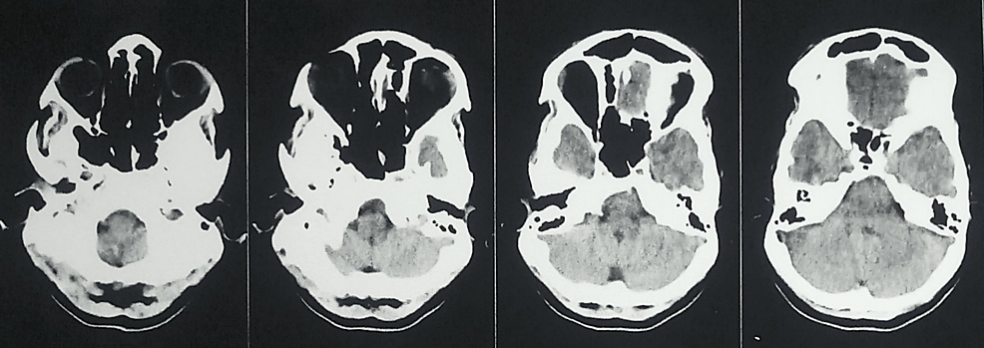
CT scan showing deviation of the mandible to the left.
